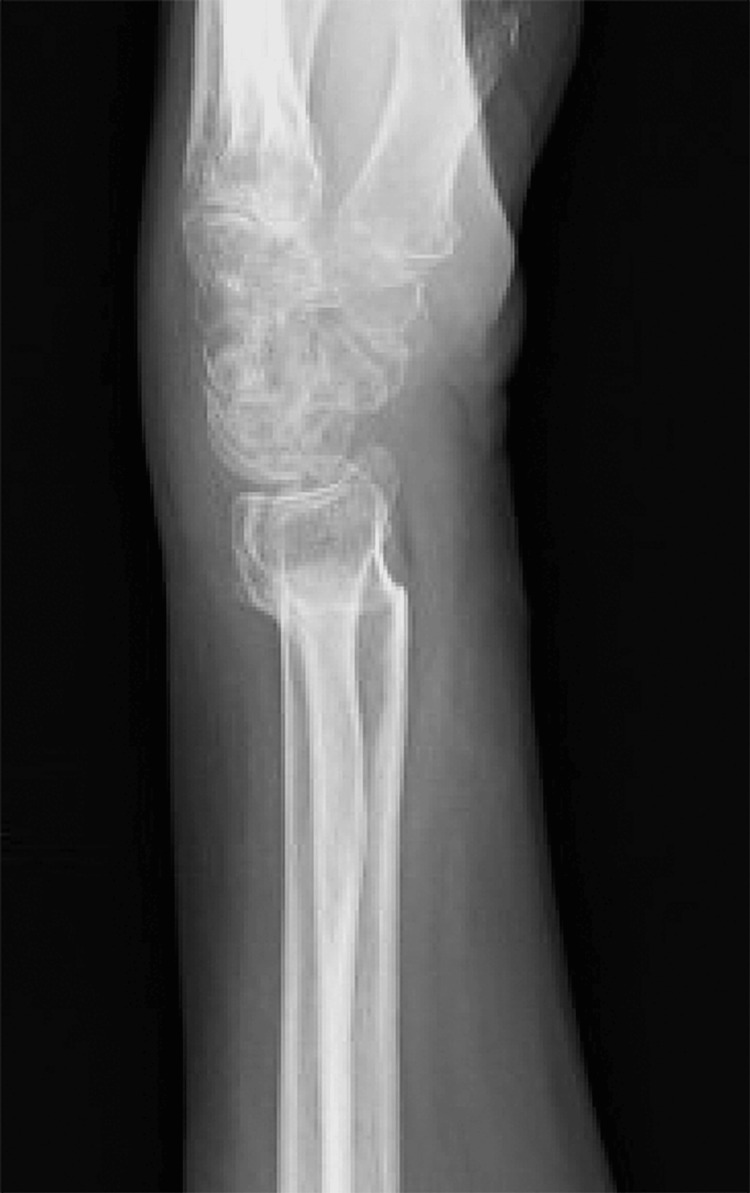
Lateral view on plain radiography 12 years earlier. Lateral view on plain radiography 12 years earlier showed a convex deformity of the volar cortex of the distal radius and a dorsal displacement (volar tilt: -10°).
